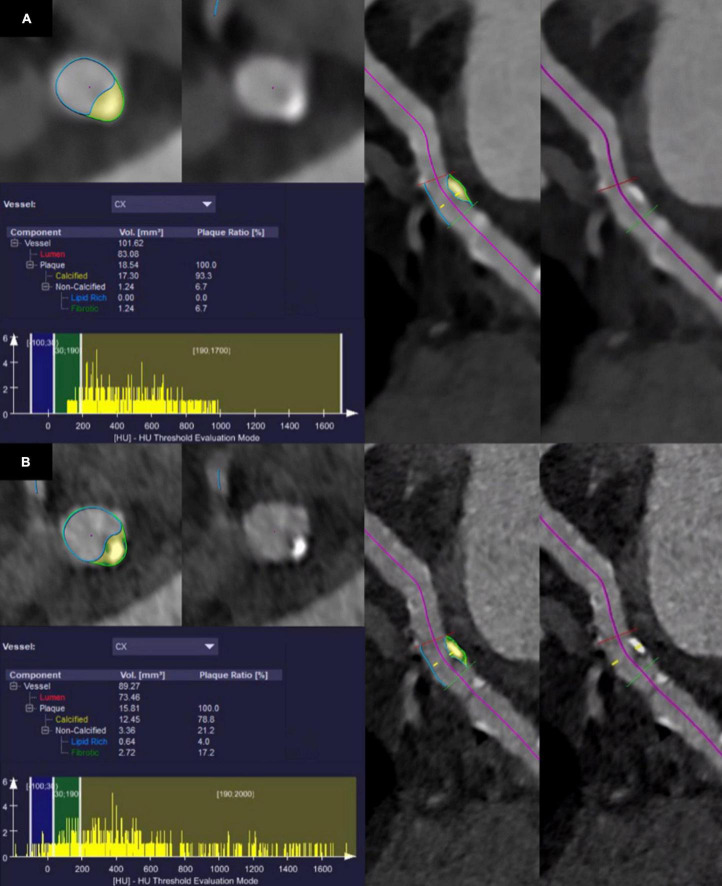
Segmentation and quantitative results of plaque characteristics in the circumflex artery of a 75-year-old male patient who underwent coronary CT angiography with photon-counting detector CT. Note the shift of CT values in the histogram towards lower values using ultra-high-resolution reconstructions with a slice thickness of 0.2 mm and the Bv64 kernel (B) compared with reconstructions with a slice thickness of 0.6 mm and the Bv40 kernel (A).
